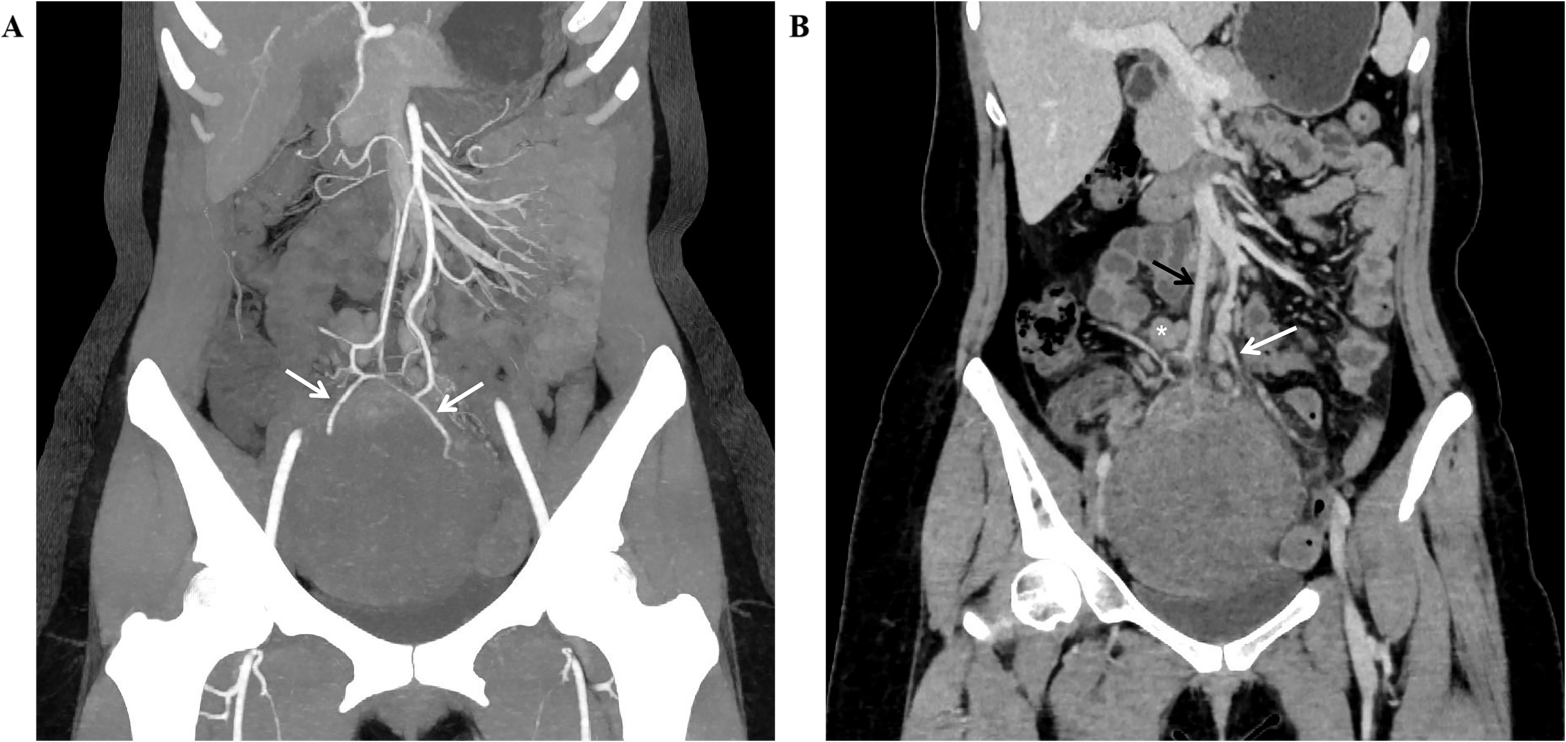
Coronal view of pelvic CT shows: (A enhanced arterial phase MIP image, B enhanced venous phase) the blood supply of the mass originates from the branches of the superior mesenteric artery (white arrow), and drains through the mesenteric vein (black arrow), with multiple enlarged lymph nodes (*) visible above the mass, A compressed small intestinal loop shadow can be observed between the mass and the bladder.
